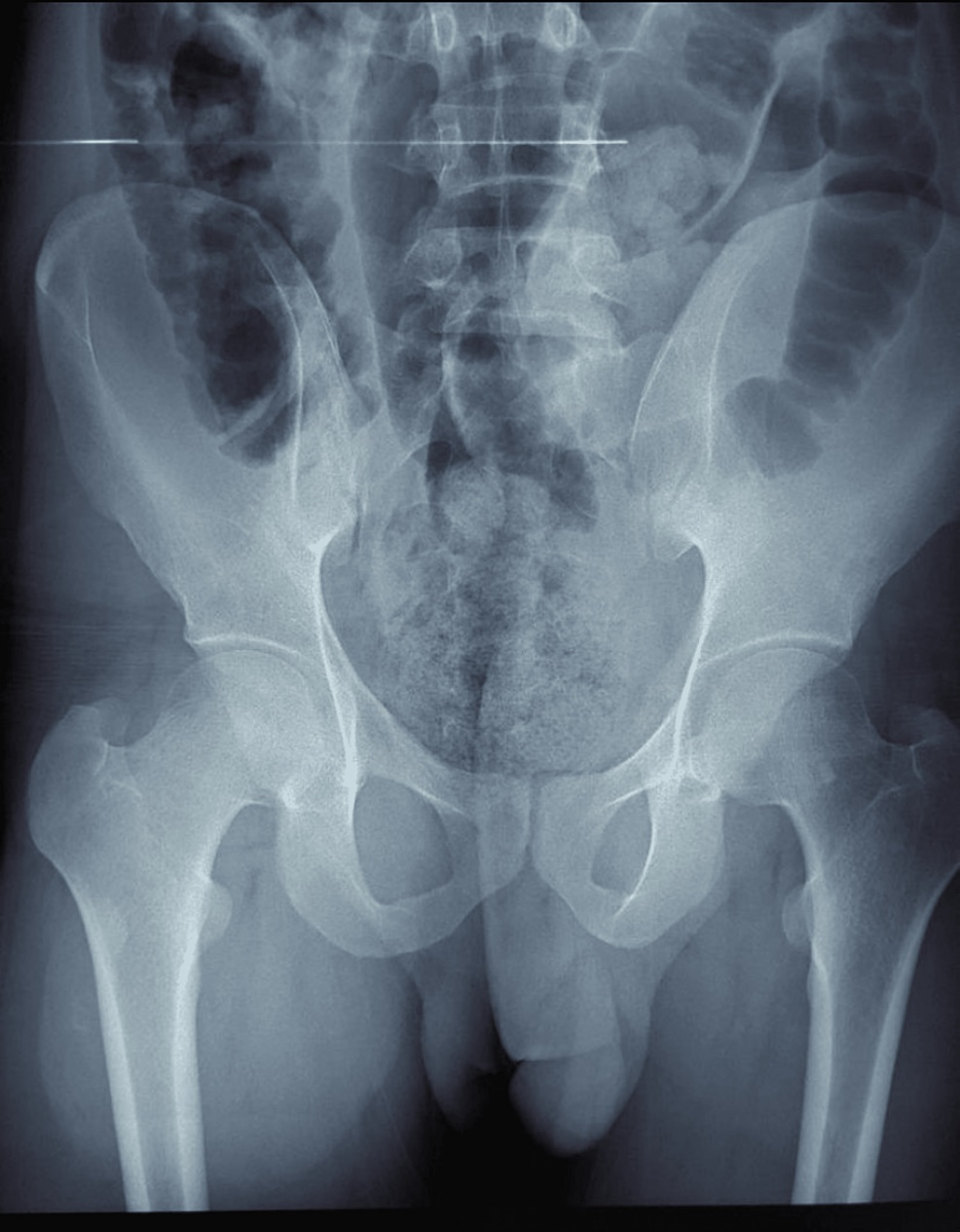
Pelvic X-ray No abnormalities were observed.
